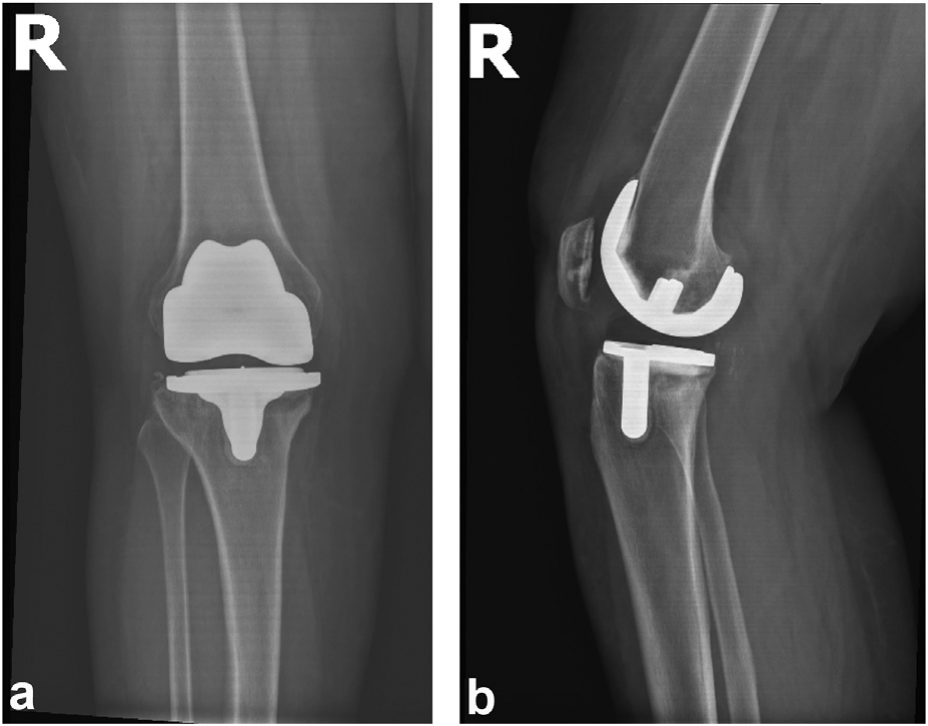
(a) Immediate postoperative AP radiograph following primary TKA obtained from outside facility 2 years prior to presentation in our clinic. (b) Immediate postoperative lateral radiograph following primary TKA demonstrating well-positioned, well-aligned cemented cruciate-retaining TKA system in place.
